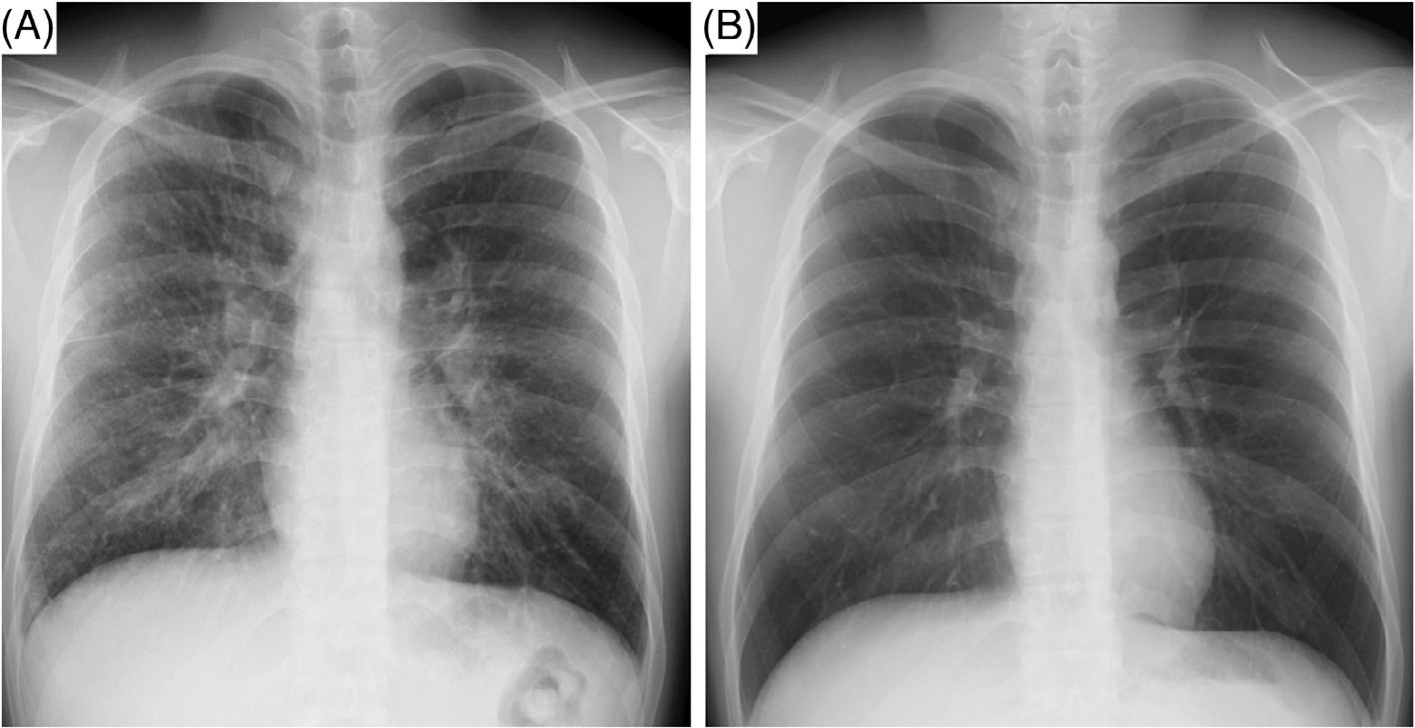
Chest radiograph on admission (A) and discharge (B). The radiograph obtained at discharge shows that the lung abnormalities had disappeared.
